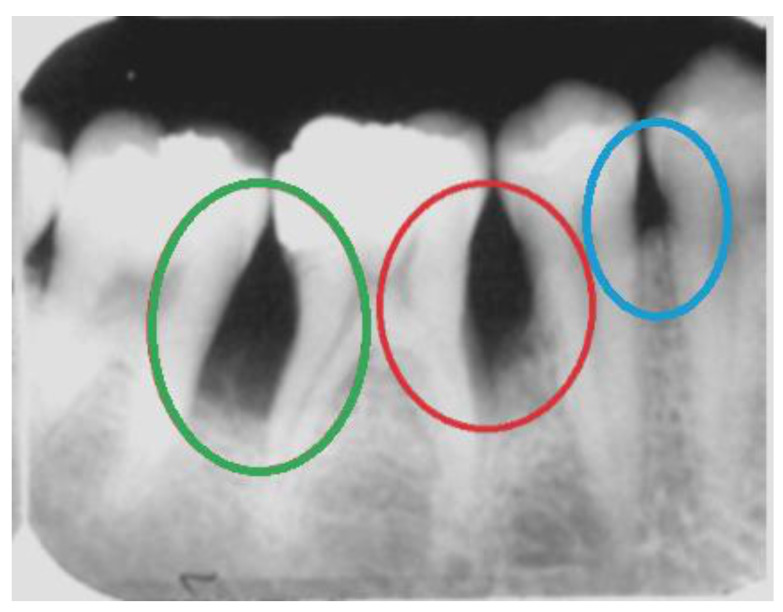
Examples of bone loss in a real periapical radiograph: an interproximal crater in green, vertical bone loss in red, and a horizontal bone defect in blue.
